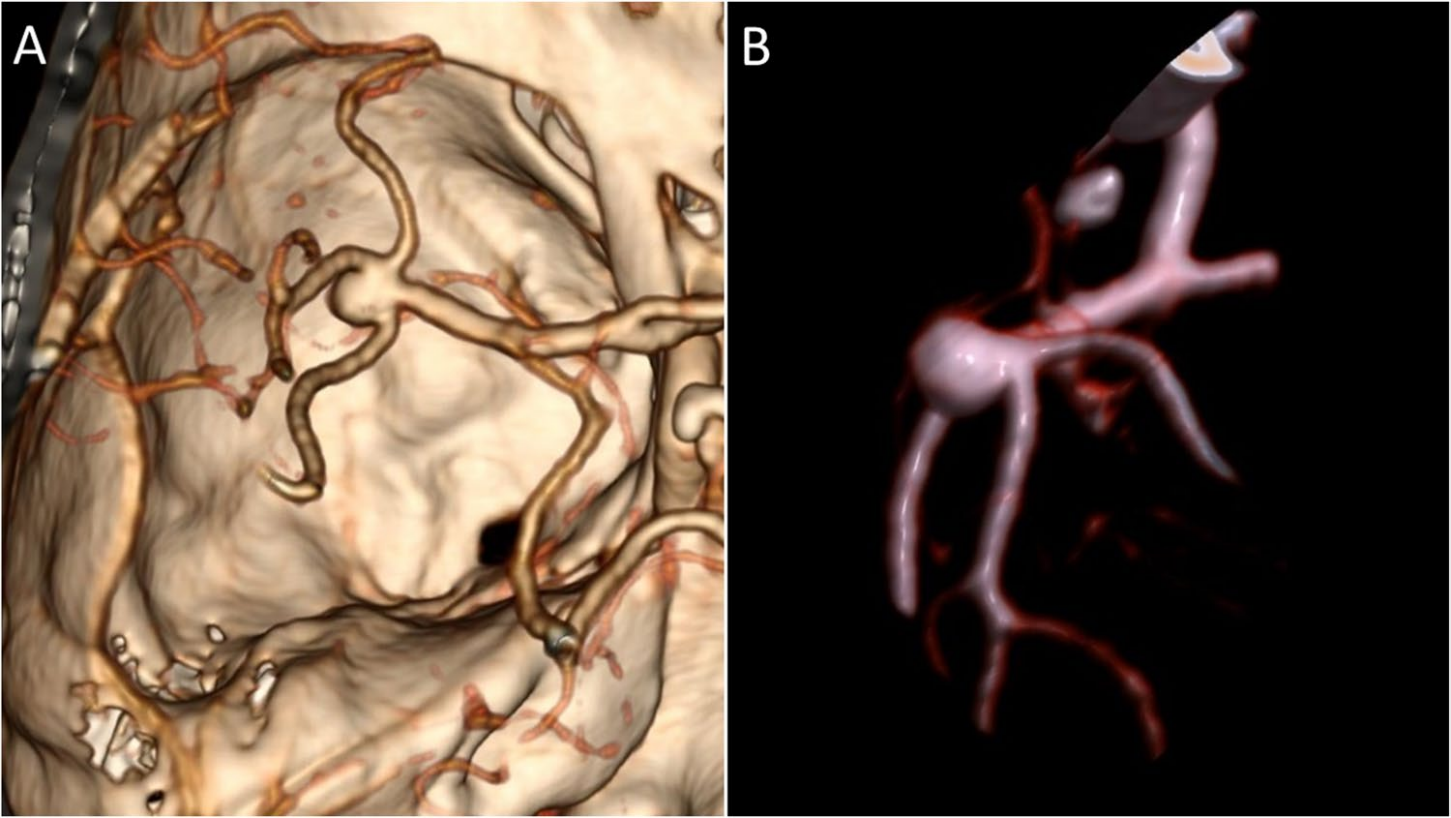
Middle cerebral artery bifurcation aneurysm with a lateral and posterior directed projection. A Three-dimensional reconstruction of CT angiographical images demonstrating the relationship of the aneurysm and its parent vessel, with surrounding bony landmarks. B Surgeon’s view of the aneurysm, M1-segmeent, and both M2 branches. An early frontal M3 branch can be appreciated. CT, computed tomography; M1, main trunk of the middle cerebral artery; M2, insular branch of the middle cerebral artery; M3, opercular segment of the middle cerebral artery
